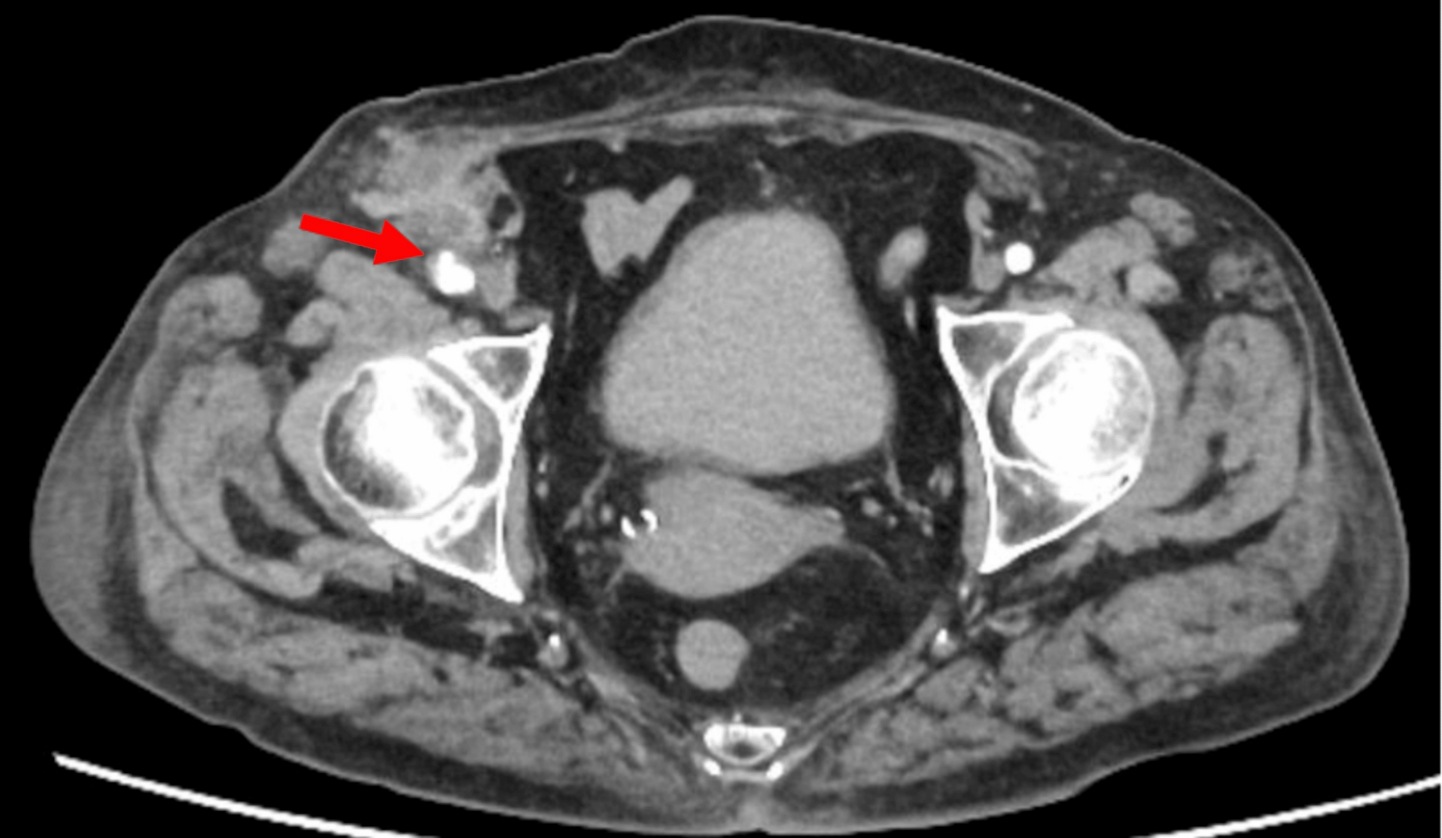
Follow-up computed tomography scan: femoral pseudoaneurysm during conservative management Follow-up contrast-enhanced computed tomography imaging showing the femoral pseudoaneurysm with a slight decrease in size and no evidence of expansion or impending rupture (arrow), supporting the decision to continue conservative management.
